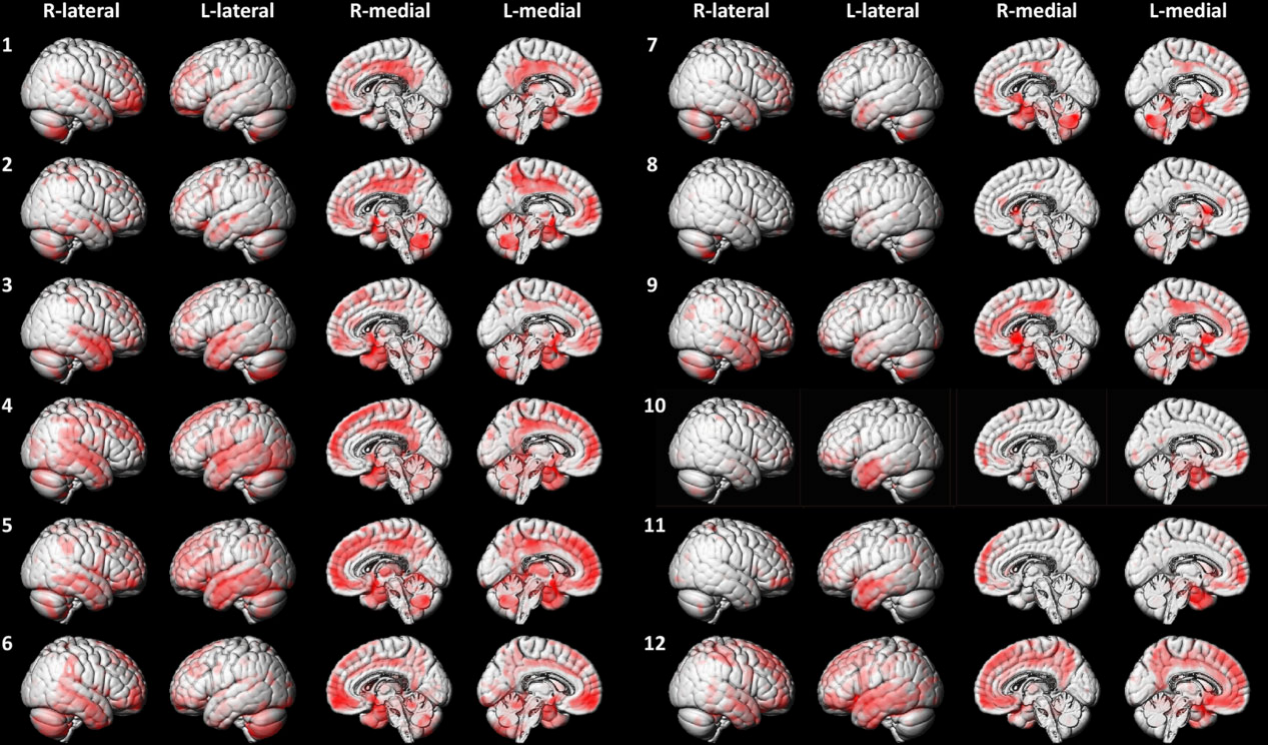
Brain MRI. Brain MRI analysed with the BAAD revealed patterns of significant atrophy in each of the 12 cases with an unclassified fluent variant of primary progressive aphasia. Case numbers are shown to the left of each set of images. Cases 1–8: progressive anomic aphasia; Cases 9–11: progressive TCSA; Case 12: progressive Wernicke’s aphasia. BAAD, Brain Anatomical Analysis using Diffeomorphic Anatomical Registration through Exponentiated Lie Algebra; L, left; R, right; TCSA, transcortical sensory aphasia.
